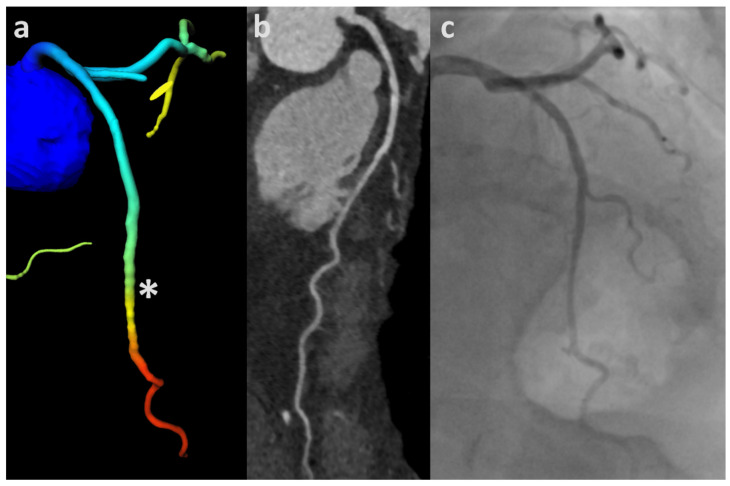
CT-FFR rendering values indicating hemodynamic significance with no apparent luminal narrowing on cCTA nor ICA: Mildly calcified left coronary artery (total CAC = 72 AU) with trifurcation into left anterior descending (LAD), left circumflex (LCX) and intermediate artery. CT-FFR values drop below 0.80 between the middle and distal segment (segment 6/7) (asterisk) (a). There is no discernable luminal obstruction on cCTA depicted as curved multiplanar reformation (b) nor on the corresponding projection of ICA (c). CAC—coronary artery calcium score; cCTA—coronary CT-angiography; CT-FFR—CT-derived fractional flow reserve; ICA—invasive coronary angiography.
